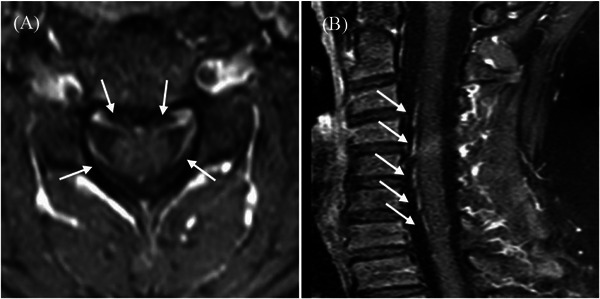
The cervical spine transverse (A) and sagittal (B) magnetic resonance imaging in patient 3 showed thickening of the spinal nerve roots with enhancement (white arrows).
